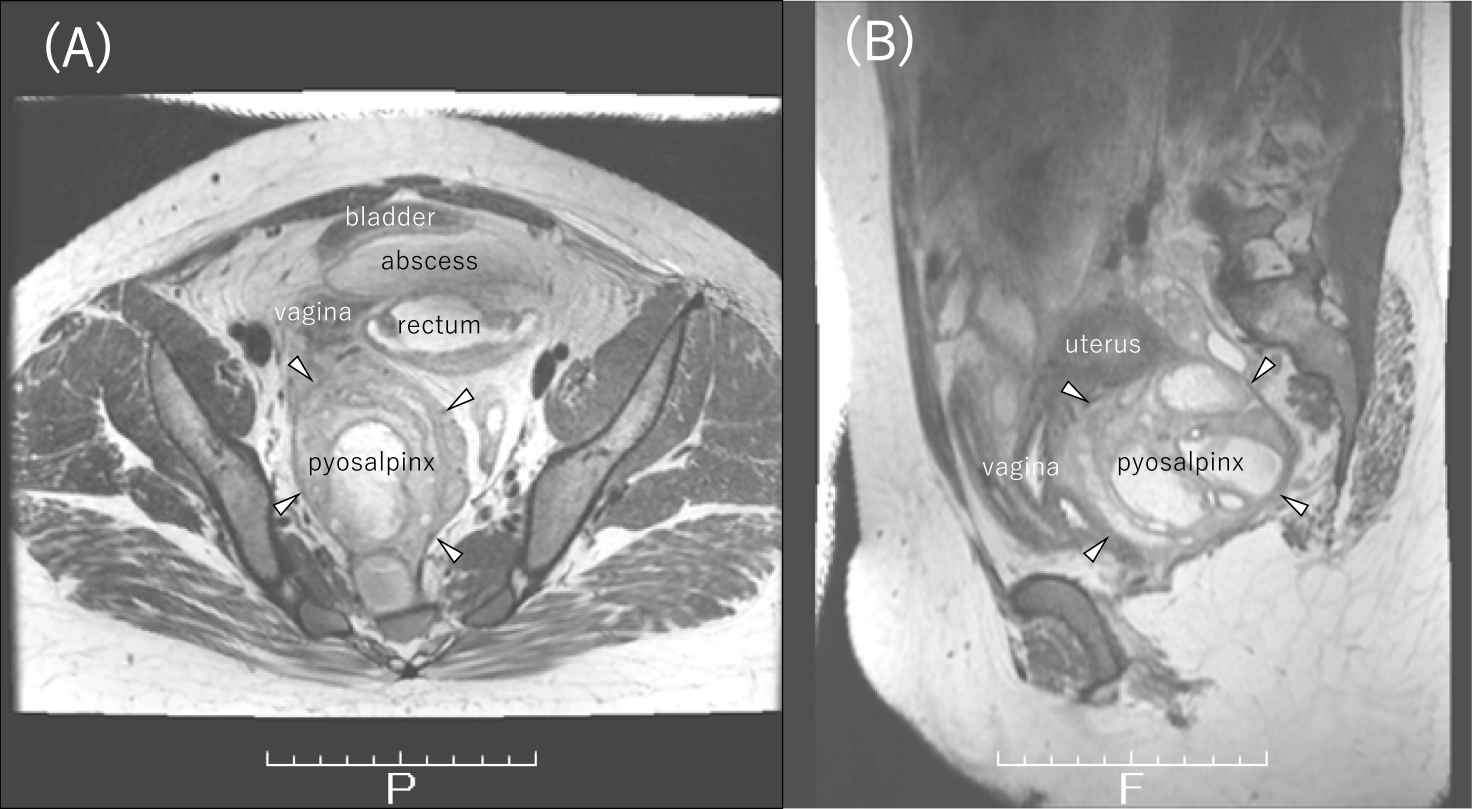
MRI. A Pyosalpinx is dorsal to the vagina and rectum (white arrowhead). The vagina is on the right side of the rectum and the abscess is between the bladder and rectum. B Pyosalpinx is dorsal to the vagina and uterus (white arrowhead)
